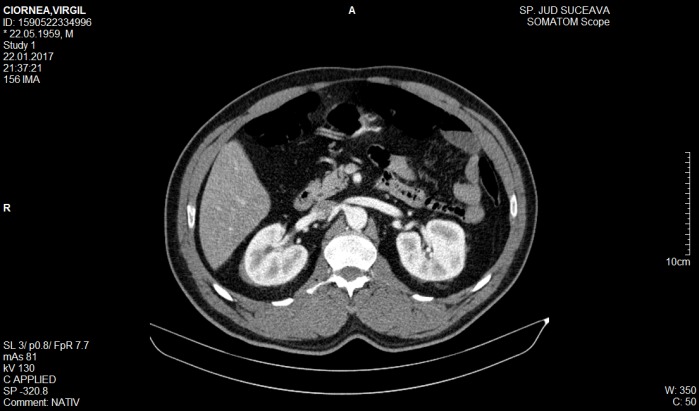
Abdominal CT - “hairy kidney”
